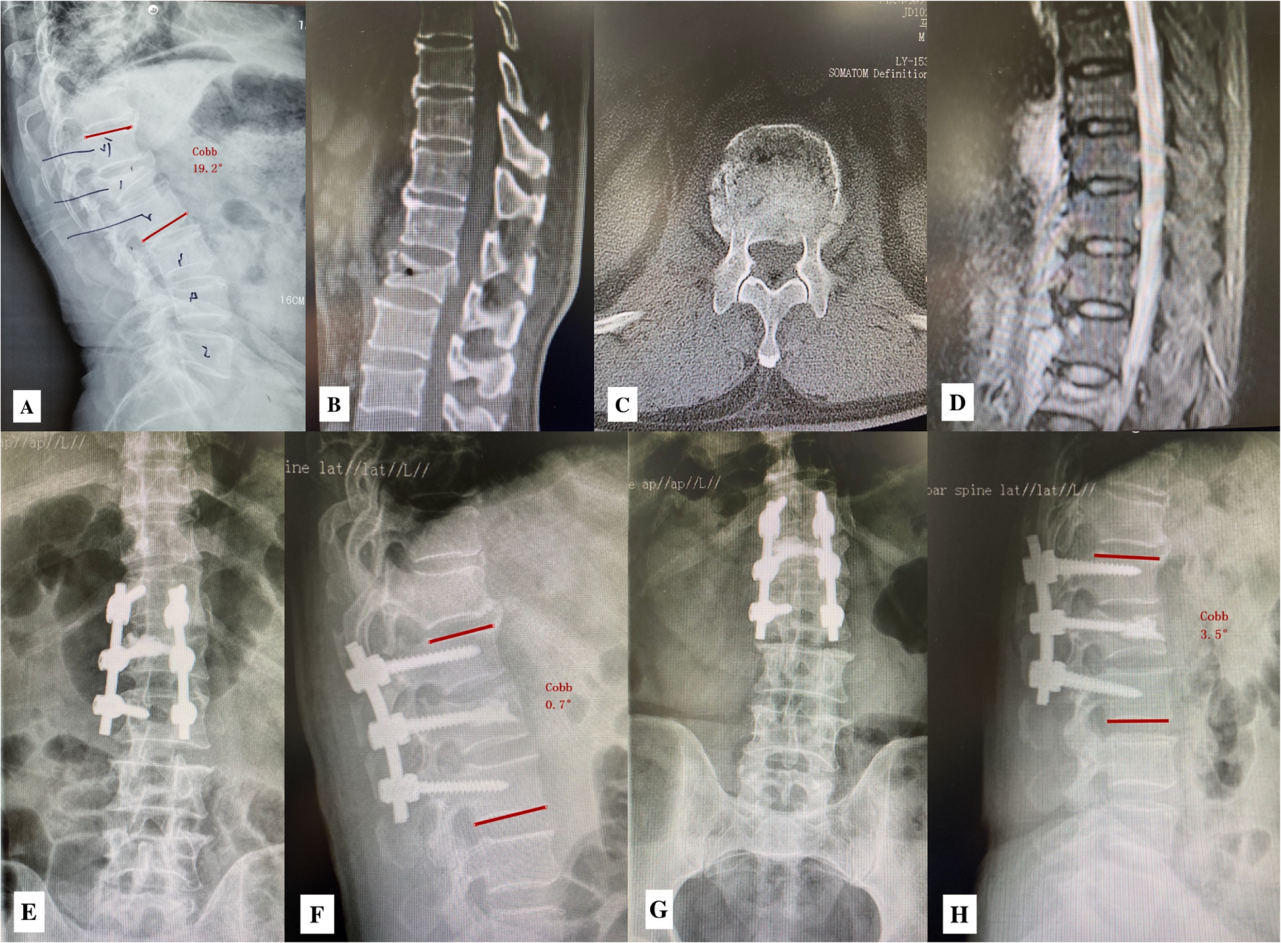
A 70-year-old male patient with L1 osteoporotic burst fracture underwent PSFFV combined with KP. a-d The preoperative X-ray, CT scan and MRI showed L1 fresh burst fracture, the Cobb angle was 19.2°, the anterior, middle and posterior vertebral height was 38, 42 and 72% respectively. e, f Postoperative X-ray showed the fractured vertebral was restored, the correction of local kyphotic angle was 18.7°, and the anterior, middle and posterior vertebral height was almost normal. g, h The final follow-up X-ray showed the correction was greatly maintained, the kyphosis was only 2.8°, and the anterior, middle and posterior vertebral height was 92, 89 and 96% respectively
